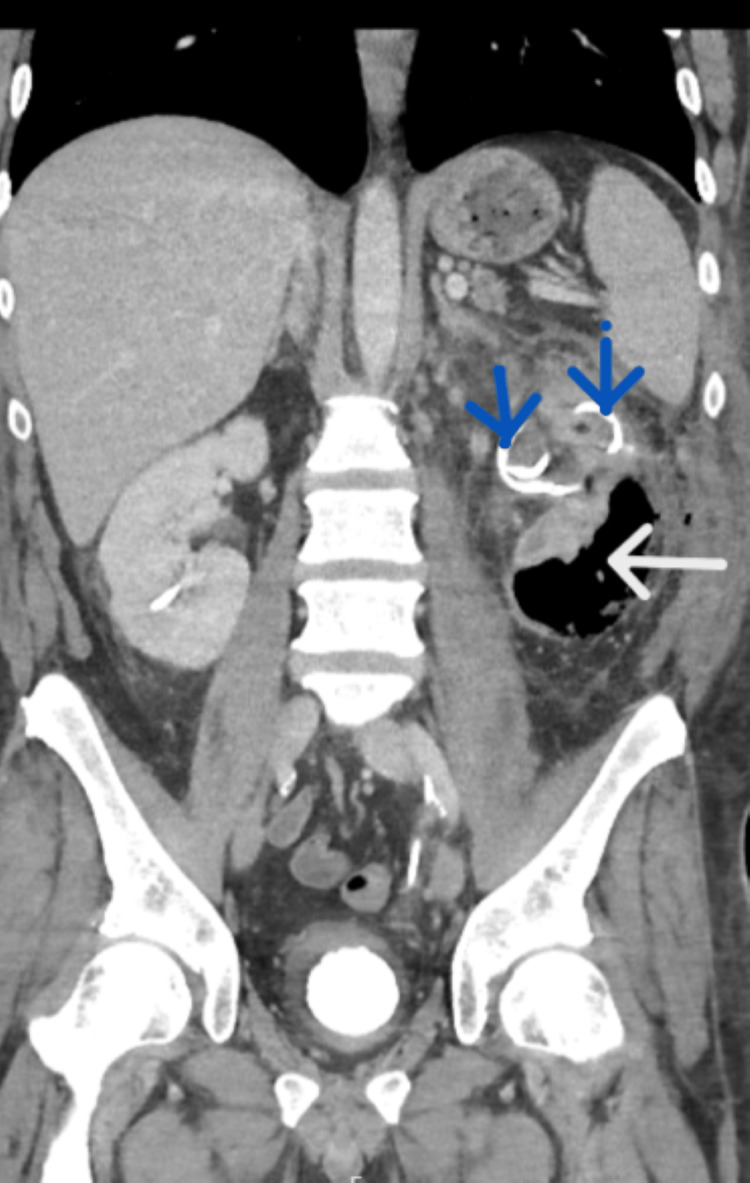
On day three of admission, a repeat coronal view of the CT scan shows the double-J ureteral stent and nephrostomy tube (blue arrows) with gas and undrained fluid in the left kidney (white arrow).
